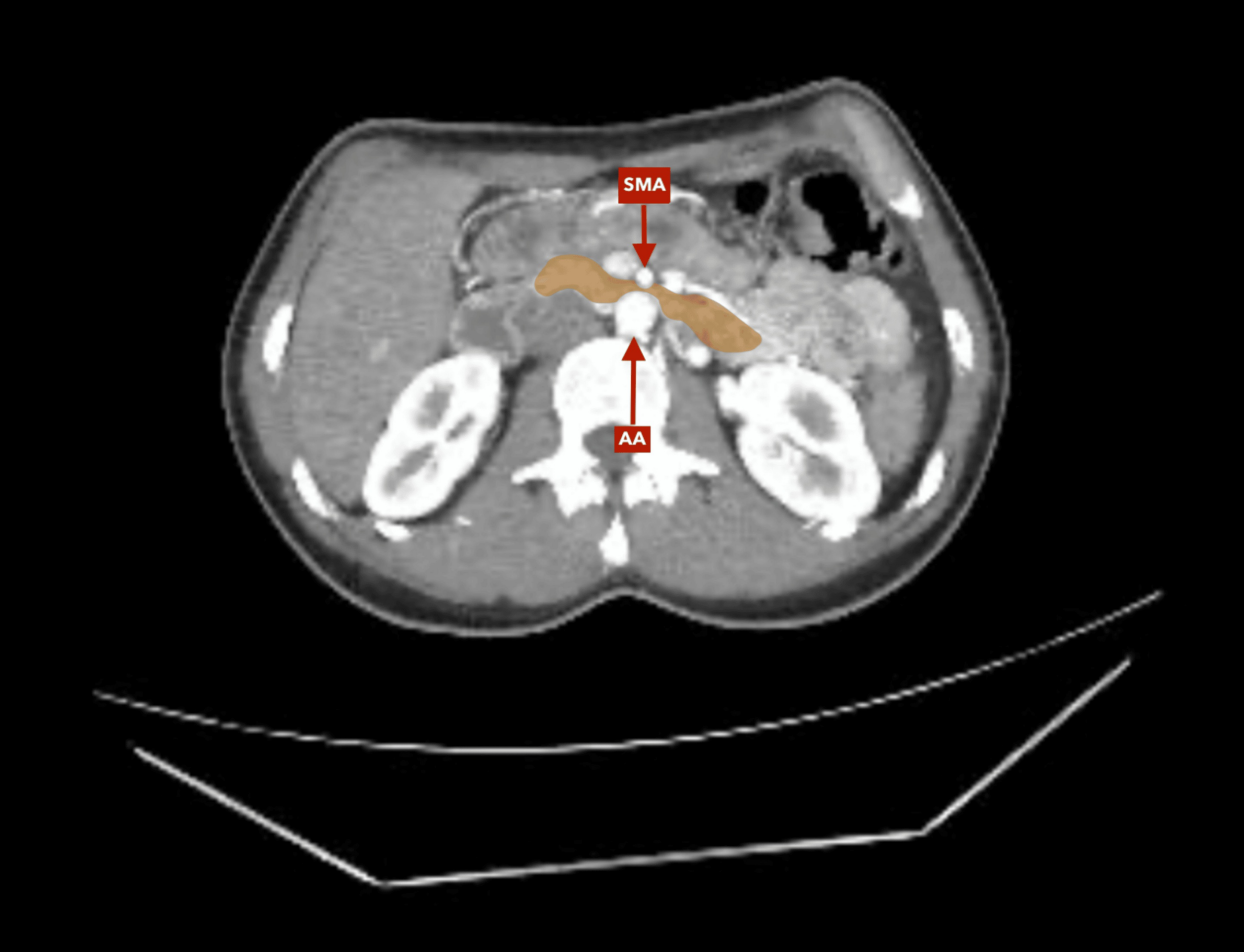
Contrast-enhanced abdominal CT scan, axial reconstruction, arterial phase. Evidence of duodenal compression by the superior mesenteric artery (SMA) on the abdominal aorta (AA). No evidence of pneumoperitoneum.
